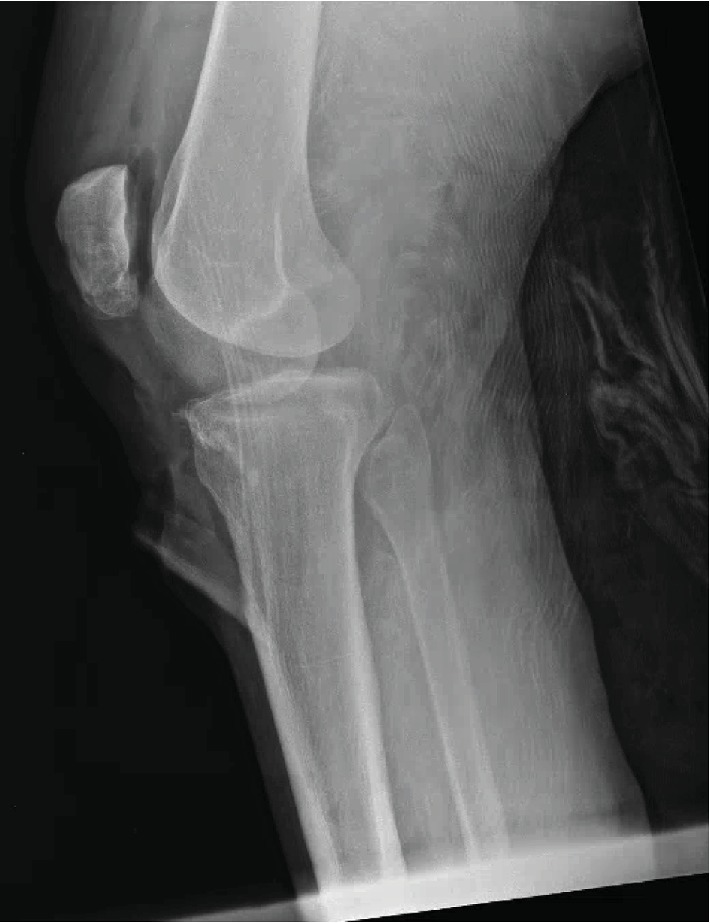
Lateral radiograph demonstrating tibial tubercle fracture and comminuted patellar fracture with overlying soft tissue defects.
